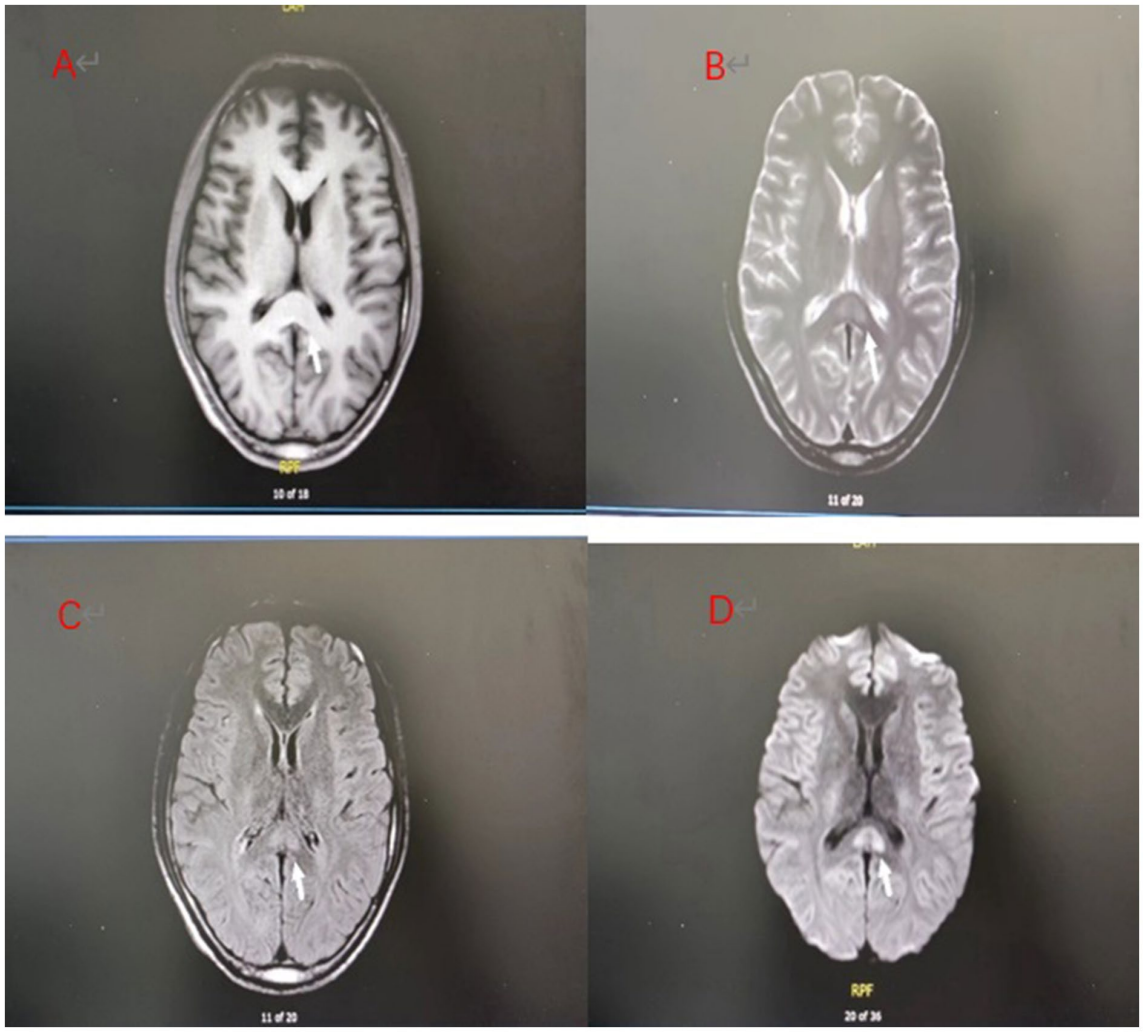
(A–D) Day 7 brain MRI:The splenial lesion of the corpus callosum had improved and might be considered as RESLES.
